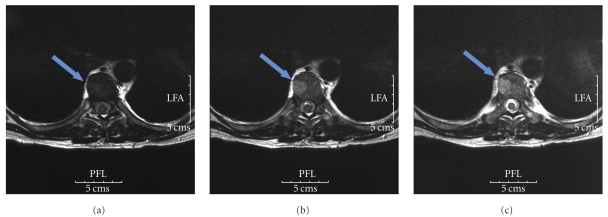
Magnetic resonance imaging of the thoracolumbar spine revealing an anterior and circular epidural mass of T8 (axial view).
